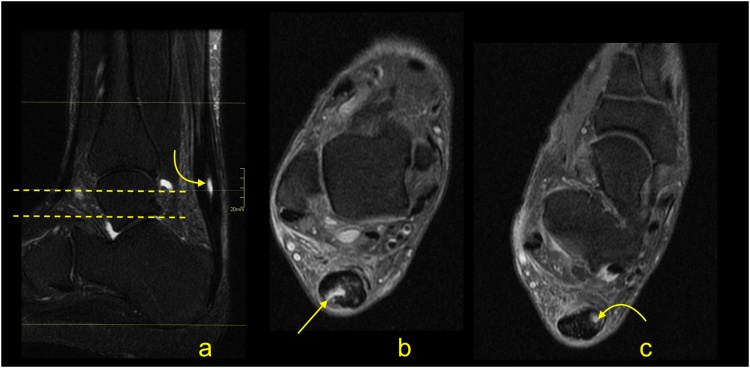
Achilles tendinopathy with intrasubstance rupture (curved arrow) and rupture on the tendon's surface (straight arrow). A 61-year-old patient with pain of the Achilles tendon for many years. A-T2-weighted with fat suppression, b and c- PD-weighted with fat suppression.
